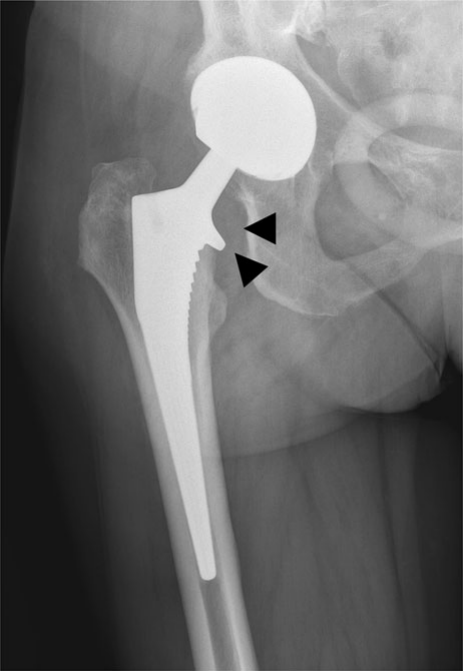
AP radiograph. Cementless total hip arthroplasty. *Arrowheads* indicating the collar of this collared femoral stem
